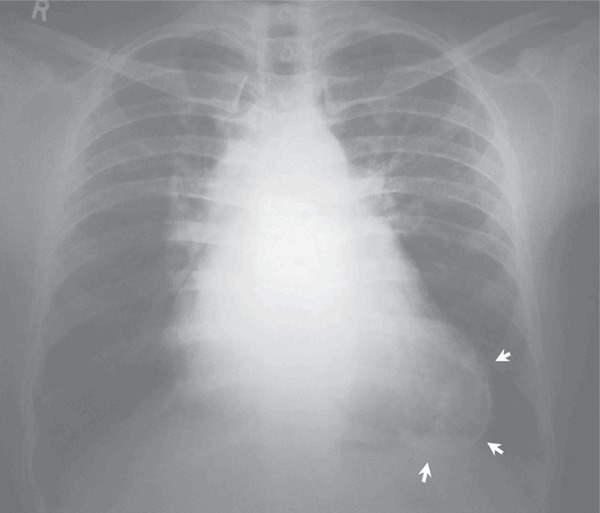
Chest X-Ray PA View Showing Calcific Left Ventricular Aneurysm (Arrows) and Features of Mitral Stenosis.
